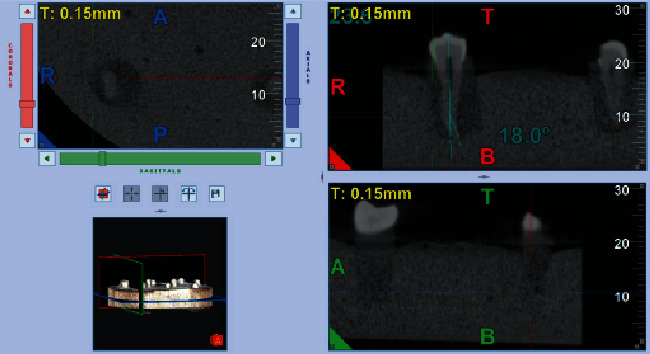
Representative CBCT image; reformatted slice showing the calibration of the root canal pathway.
